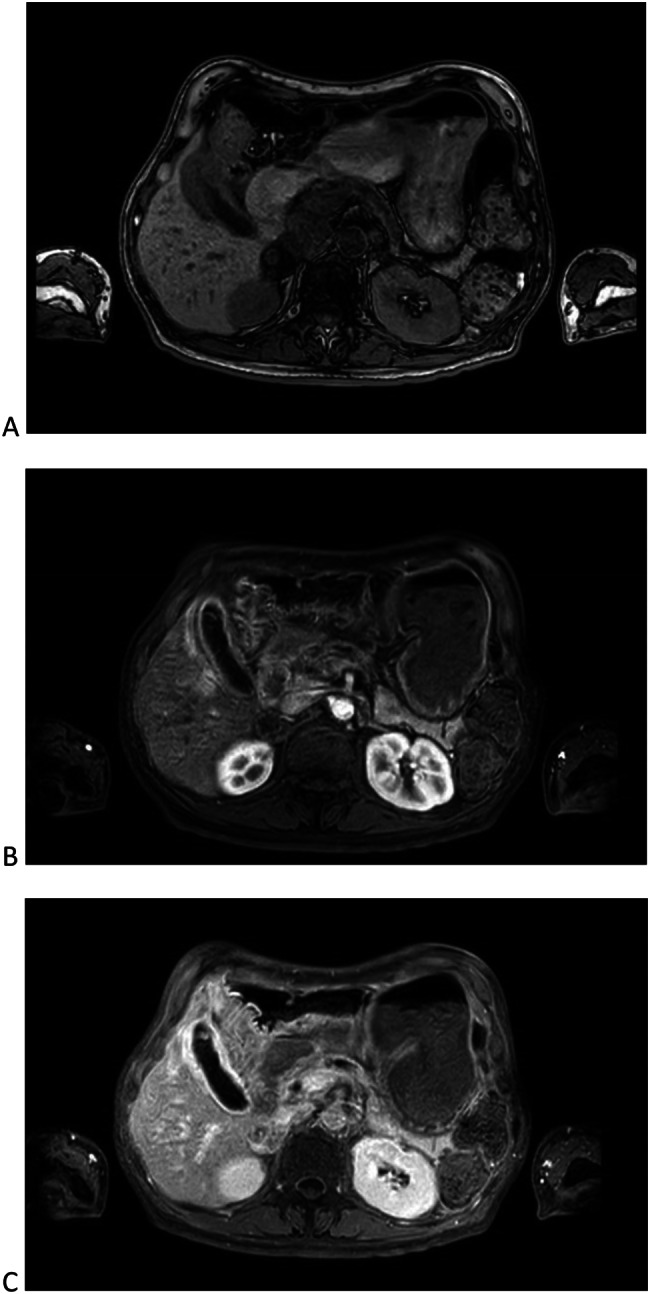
Abdomen MRI findings: A T1-weighted sequence showing irregular thickening of the distal body and fundus of the gallbladder wall (maximum diameter 18 mm). The thickness was T1 hypo-intense to the surrounding liver. B Early arterial enhancement of the wall thickening after contrast agent injection, with reactive hyperemia of the adjacent liver parenchyma. C) Venous phase documents retention of the contrast agent in venous phase
